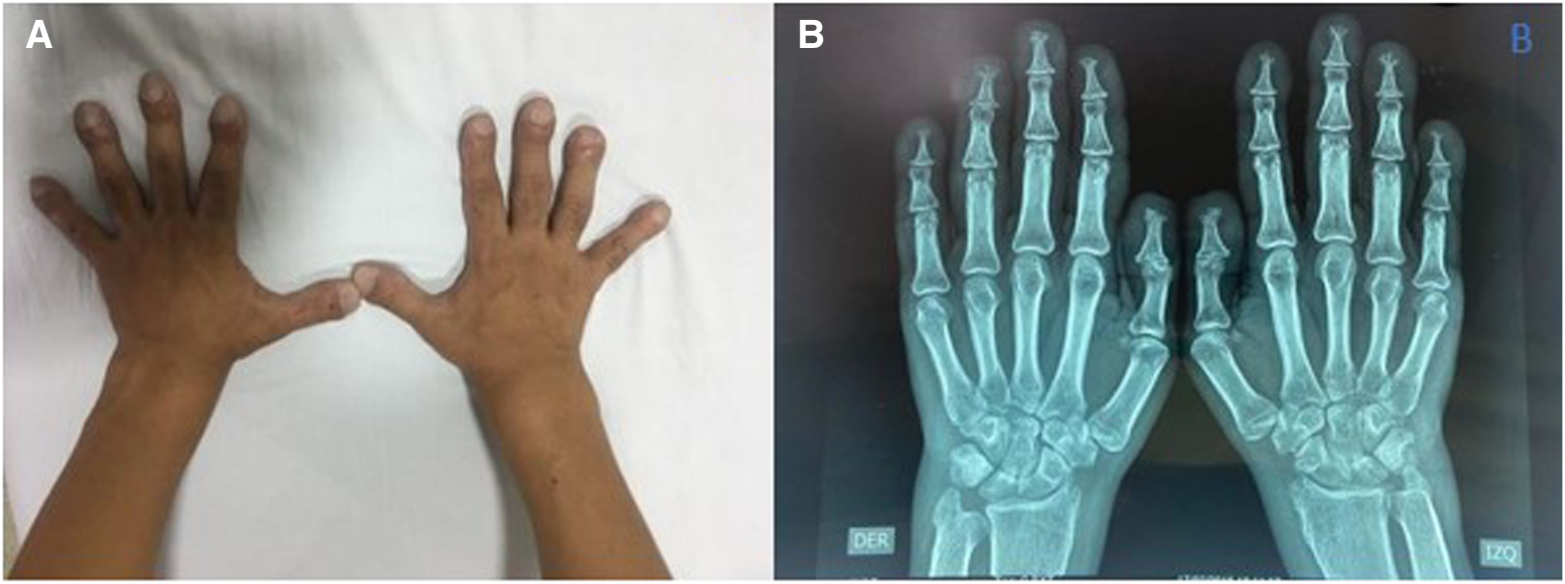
(*A*) Clubbed fingers with distal thumb deviation. (*B*) Anteroposterior X-ray of both hands show bone resorption of the distal phalanges (acro-osteolysis).
